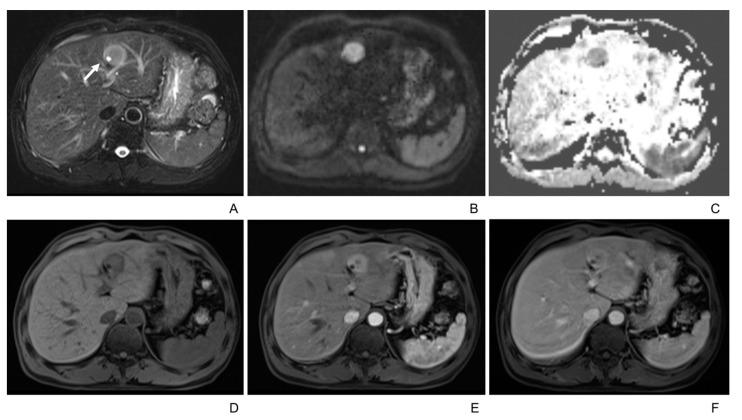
49-year-old male with intrahepatic lymphoepithelioma-like cholangiocarcinoma. (A) T2-weighted MR image displays a well-defined hyperintense tumor in the left lobe of the liver, with hyperintense foci inside it (white arrow). (B,C) Tumor shows hyperintensity on diffusion-weighted imaging (b = 500 m^2^/s) and hypointensity on the ADC map. (D–F) Tumor shows hypointensity in plain scan and rapid wash-in without wash-out (Type C enhancement pattern) in the arterial and portal venous phase.
